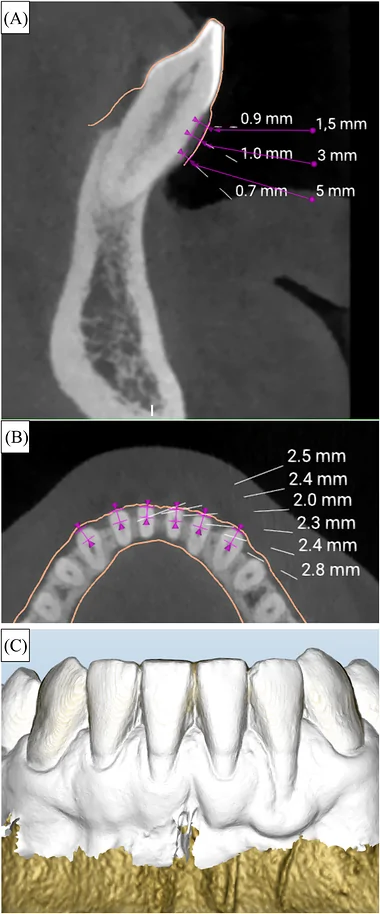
Radiographic measurements using cone‐beam computed tomography (CBCT). (A) Gingival thickness measured at 1.5, 3, and 5 mm from the gingival margin. (B) Root prominence assessment. (C) Superimposition of standard tessellation language (STL) and Digital Imaging and Communications in Medicine (DICOM) files for integrated digital analysis.
